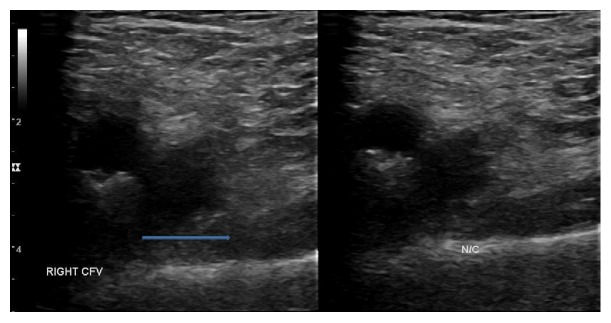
Noncompressibility of right common femoral vein (CFV) seen on duplex ultrasound.
